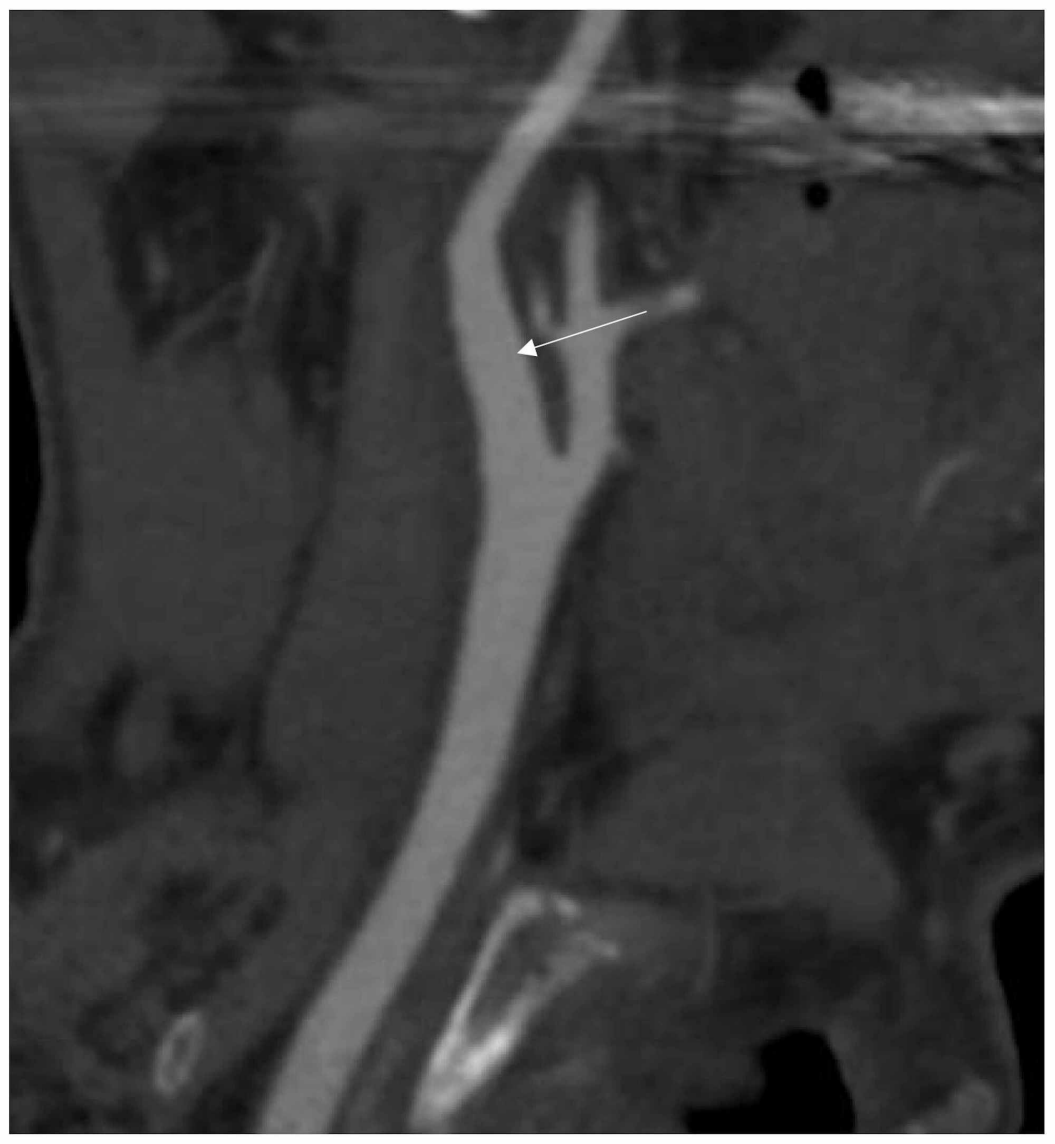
Computed tomography angiography follow-up of case 1 Computed tomography angiography sagittal view on 24-day follow-up showing complete resolution of the carotid plaque
